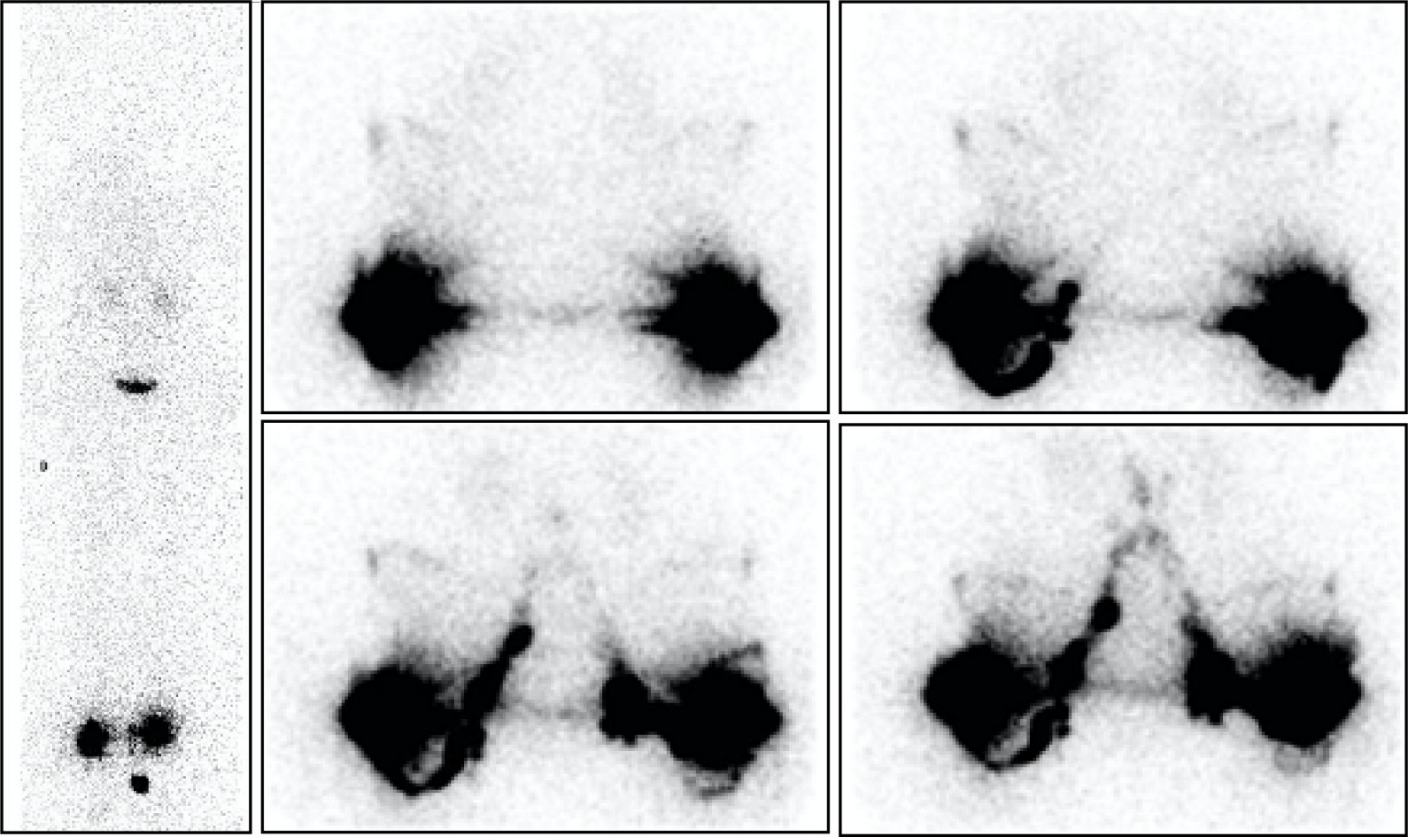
“No LNs observed in one case of primary bilateral LLLE? They are present!”. Phase 3 lymphoscintigraphy [on the left: Anterior WBS] in a 70-year-old woman with primary left-sided LLLE for 30 years showed no lymphatic vascular drainage and no infra-diaphragmatic LNs on either side. However, intradermal injections performed on the same day in the external part of the buttocks revealed LVs coursing in the direction of the right inguinal area, reaching the inguinocrural LNs and thereafter the iliac and lumbo-aortic LNs, bilaterally [on the right: Four consecutive anterior views, from left to right and top to bottom, centered on the pelvis and abdomen].
